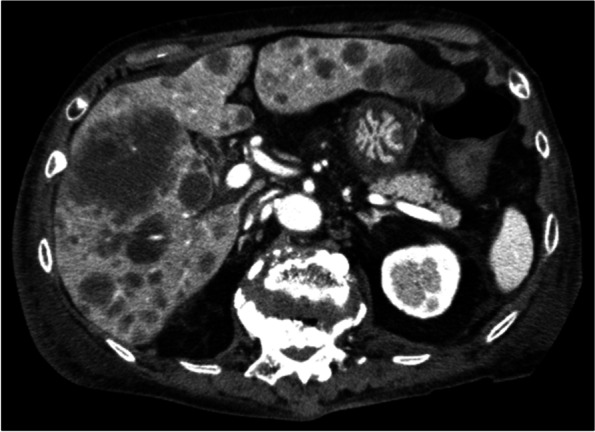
Multiple hepatic nodules were detected in a patient with systemic EBV+ CD8+ TCL (Case No. 13) by computed tomography. The maximum nodule size was 78 mm, and no lymphadenopathy was found in the abdomen
